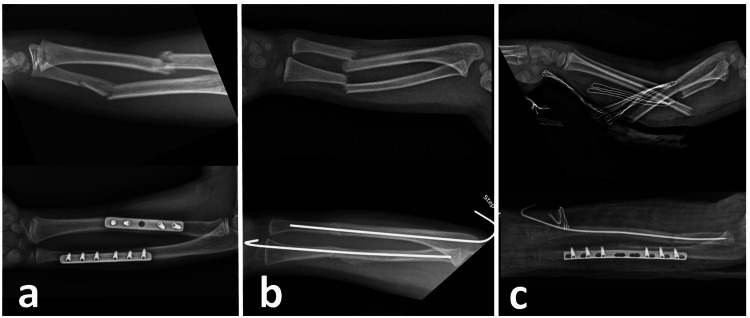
Pre-operative and post-operative follow-up radiographs of three types of fixation techniques. a) A-11-year-old boy with forearm double fractures treated with plate-screw fixation b) A-8-year-old girl with forearm double fractures underwent ESIN fixation. c) A-10-year-old boy with forearm double fractures operated with hybrid fixation.
